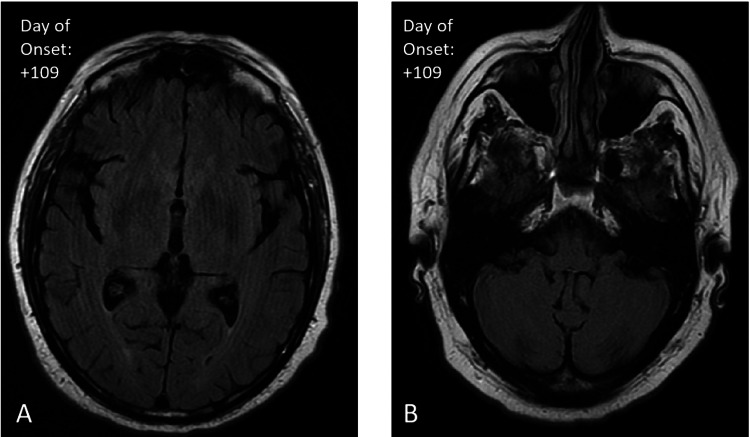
Post-treatment axial T2 FLAIR MRI. Day 109 after the onset of symptoms showing complete resolution of the previously observed right occipital (A) and left cerebellar abnormalities (B). FLAIR: fluid-attenuated inversion recovery.
